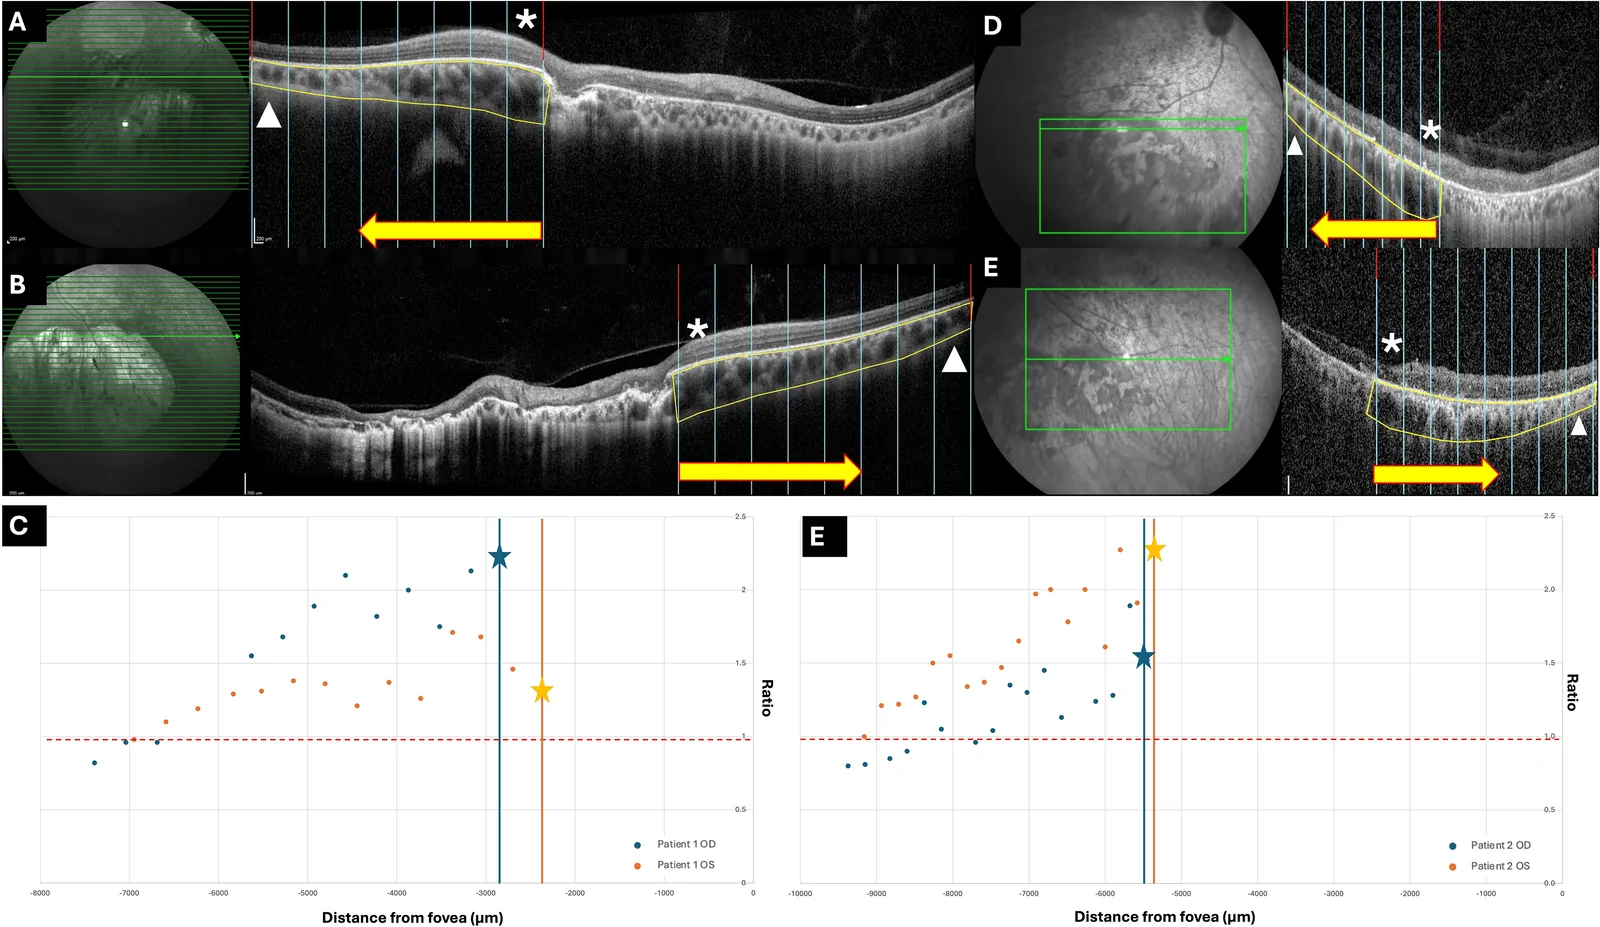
Ratio assessment of choroidal thickness adjacent to chorioretinal dysplasia. In patient 1, the region of interest (ROI) adjacent to chorioretinal dysplasia was segmented into eight equidistant sections in the right (A) and left (B) eyes. Choroidal thickness (CT) adjacent to the dysplastic border (asterisk) was expressed as a ratio relative to the most temporal measurement (arrowhead) and mapped according to distance from the fovea (C). Corresponding analysis was performed in patient 2 in the right (D) and left (E) eyes, with ratios mapped in (F). Blue and orange vertical lines denote the initiation point of chorioretinal dysplasia in the right and left eyes, respectively
